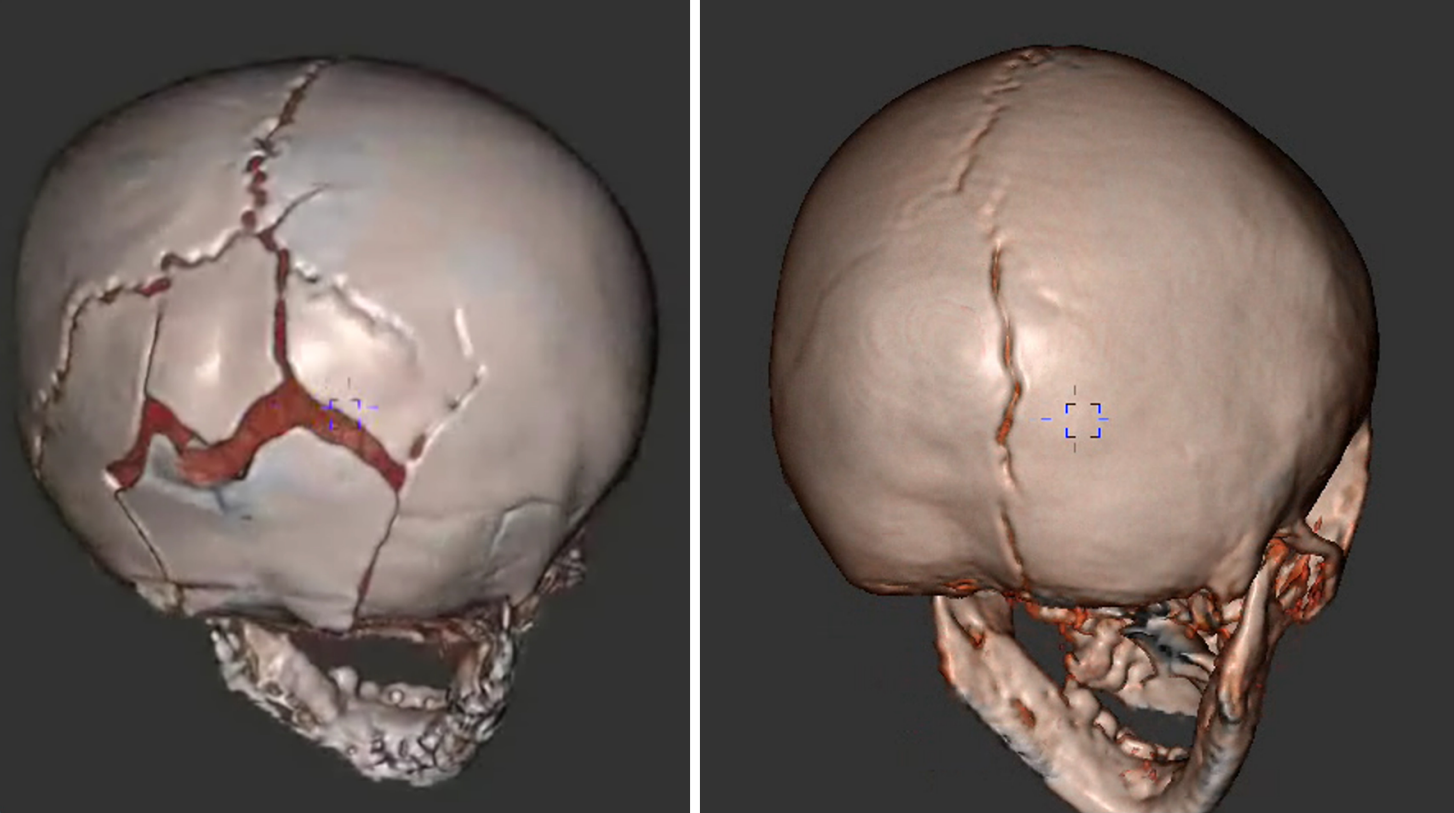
Semi-automated 3D segmentation of PETRA imaging in infants with skull fractures secondary to abusive head trauma using Fovia High-definition volume rendering software (MRI data acquisition courtesy of Dr S Kralik)
